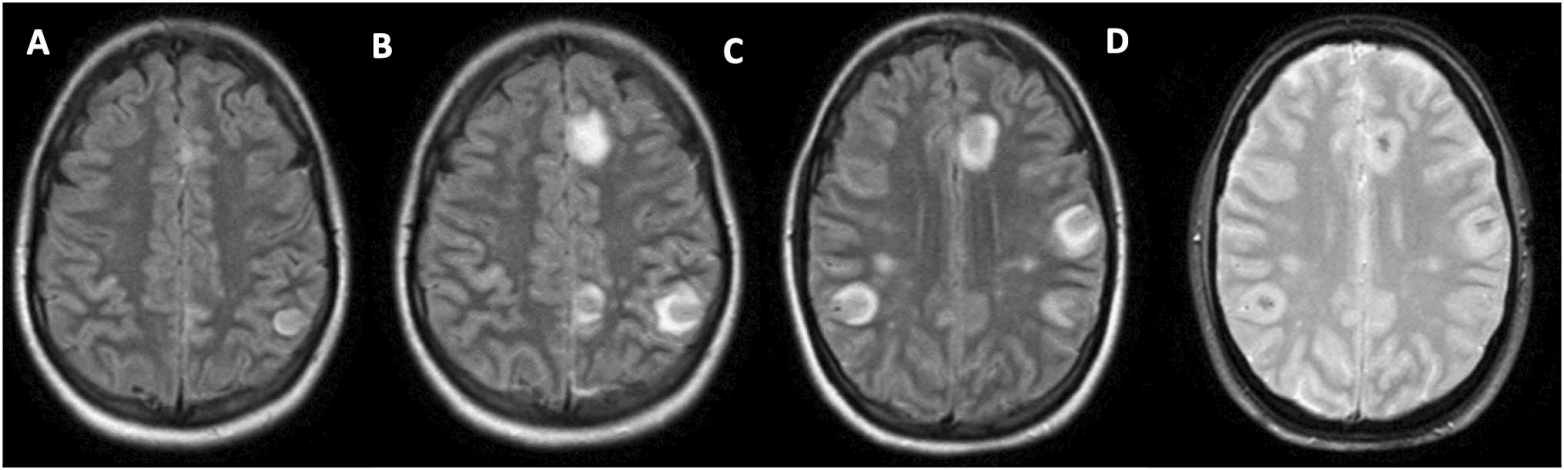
Brain MRI. Brain MRI 1 week after symptom onset showed multiple T2-FLAIR hyperintense lesions particularly located at the supratentorial gray-white junction (A). MRI 3 days later revealed a massive worsening of the imaging findings with an increase of the lesions both in size and number (B, C), now also showing central hemorrhagic transformation (D, T2*: dark intralesional spots)
